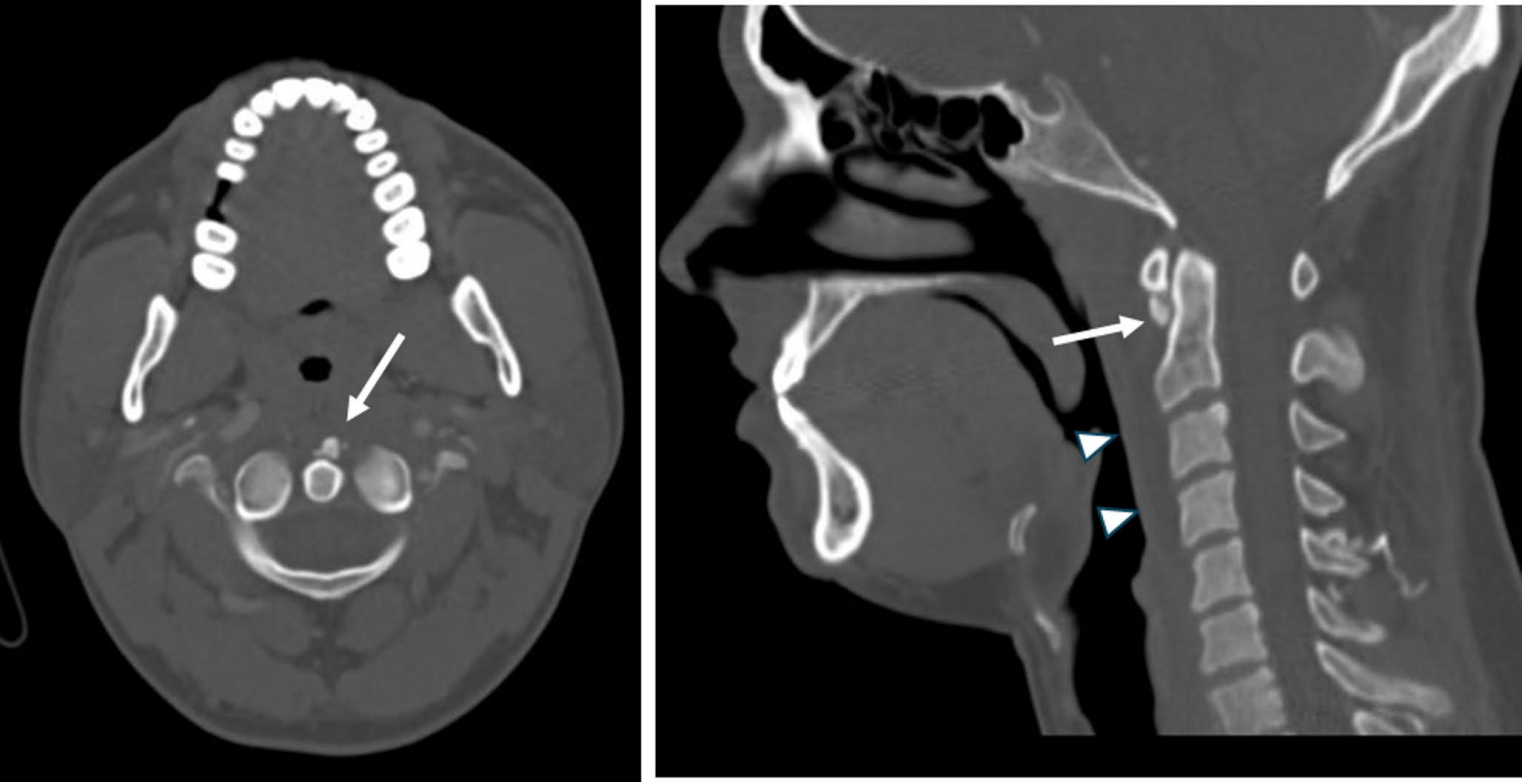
Left: Axial CT of a patient with a clear calcification on the anteroinferior border of the anterior arch of C1 (white arrow). Right: On Sagittal CT there is also a thin hypodense tissue (white arrow heads) that runs from C1 to C5 corresponding to retropharyngeal edema
